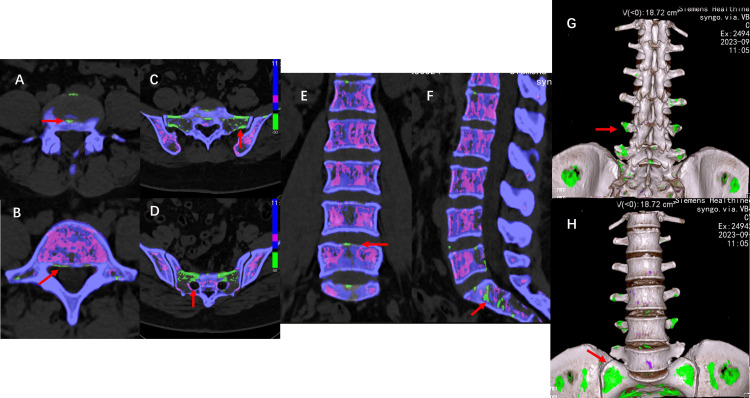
Dual-energy CT at presentation (A) There are deposits in the L4-L5 intervertebral disc; (B) There are deposits on the posterior edge of the L5 vertebra and on the bilateral transverse processes; (C) There are deposits on the S1 vertebra and the bilateral sacral wings; (D) There are deposits surrounding the bilateral sacral foramina; (E) Coronal images reveal deposits along the margins of the vertebra; (F) Sagittal images reveal deposits along the edges of the lumbar and sacral vertebrae, as well as within the sacral vertebrae; (G) 3D posterior aspect of the lumbar spine; (H) 3D Anterior aspect of lumbar spine. The green area indicated by the red arrow shows the urate deposits, with a volume of 18.72 cm³.
